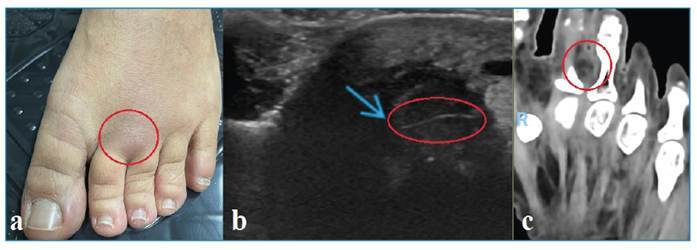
(a) Appearance of a foot lesion showing edema and erythema. (b) Demonstration of larva migrans as a hyperechoic band on ultrasound. (c) Demonstration of a hypointense area with hyperintense debris on magnetic resonance imaging.
